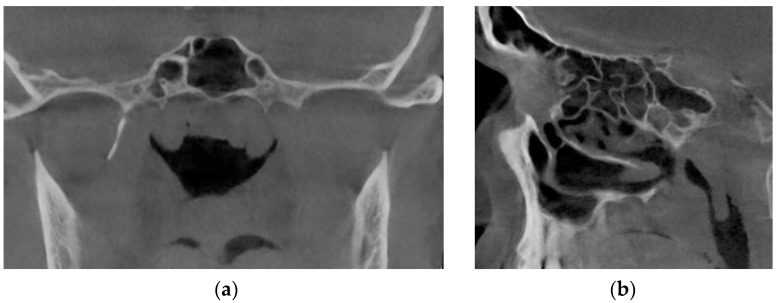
Computed tomography of a patient with adenoid vegetation: (a) axial tomographic section; (b) multiplanar reconstruction in sagittal projection.
